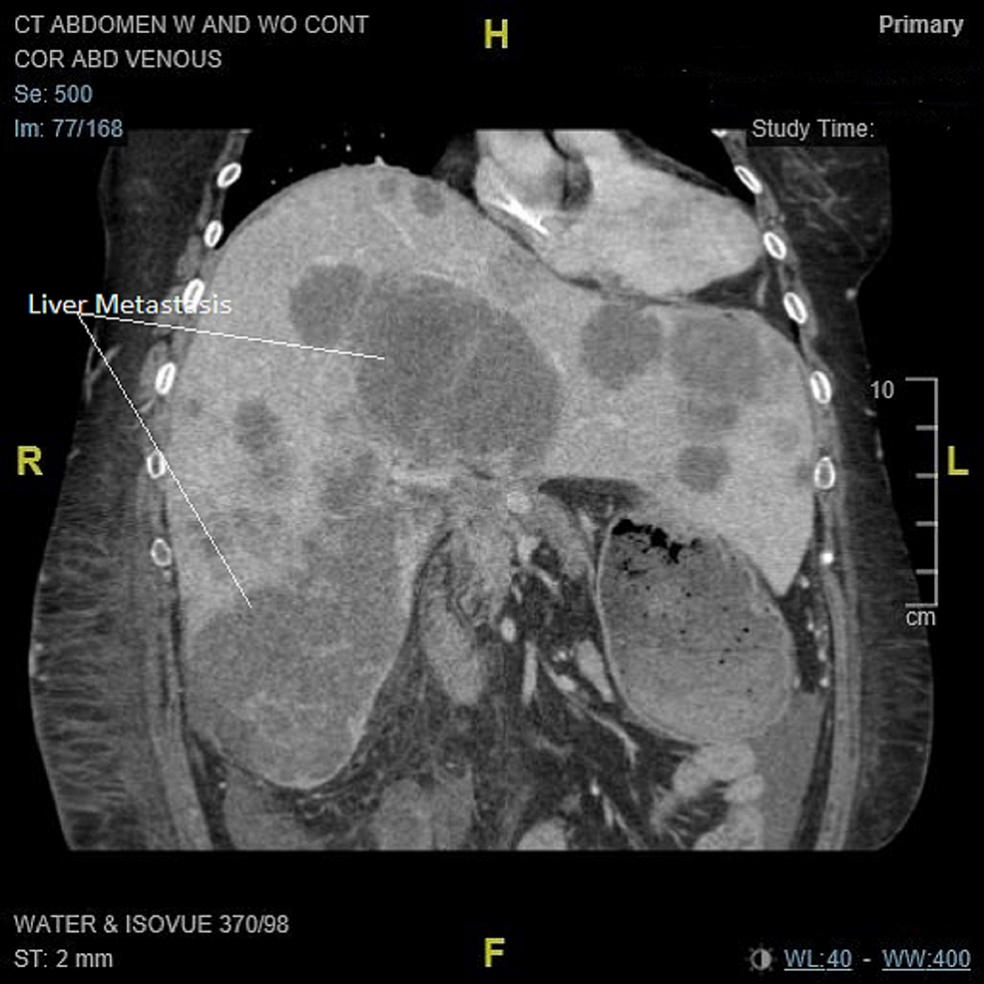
CT abdomen (coronal plan): multiple liver metastasis.
